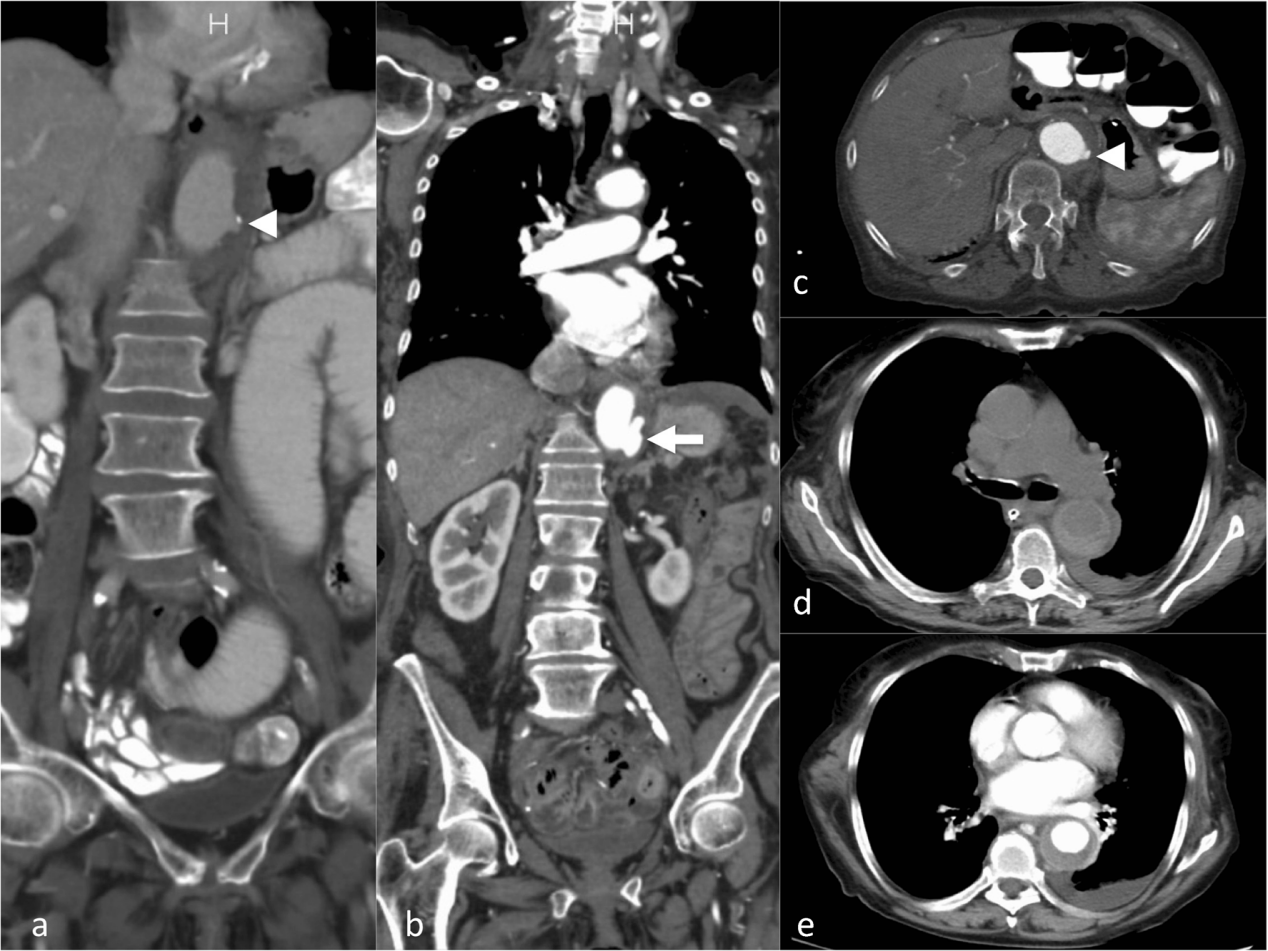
Intramural haematoma in a 79-year-old female patient. The patient presented with thoracic pain. Initial CTA with coronal reformations (a) and axial section (c) CTA demonstrated a small ulcer-like configuration (*arrowhead*) in the intramural haematoma that ascended up to the left subclavian artery (a). One-week follow-up CTA demonstrated enlargement of the ulcer-like configuration (*arrow*) (b). Native CT demonstrates the hyperdense intramural haematoma (d) and contrast-enhanced CT the smooth circumferential configuration of the intramural haematoma (e)
